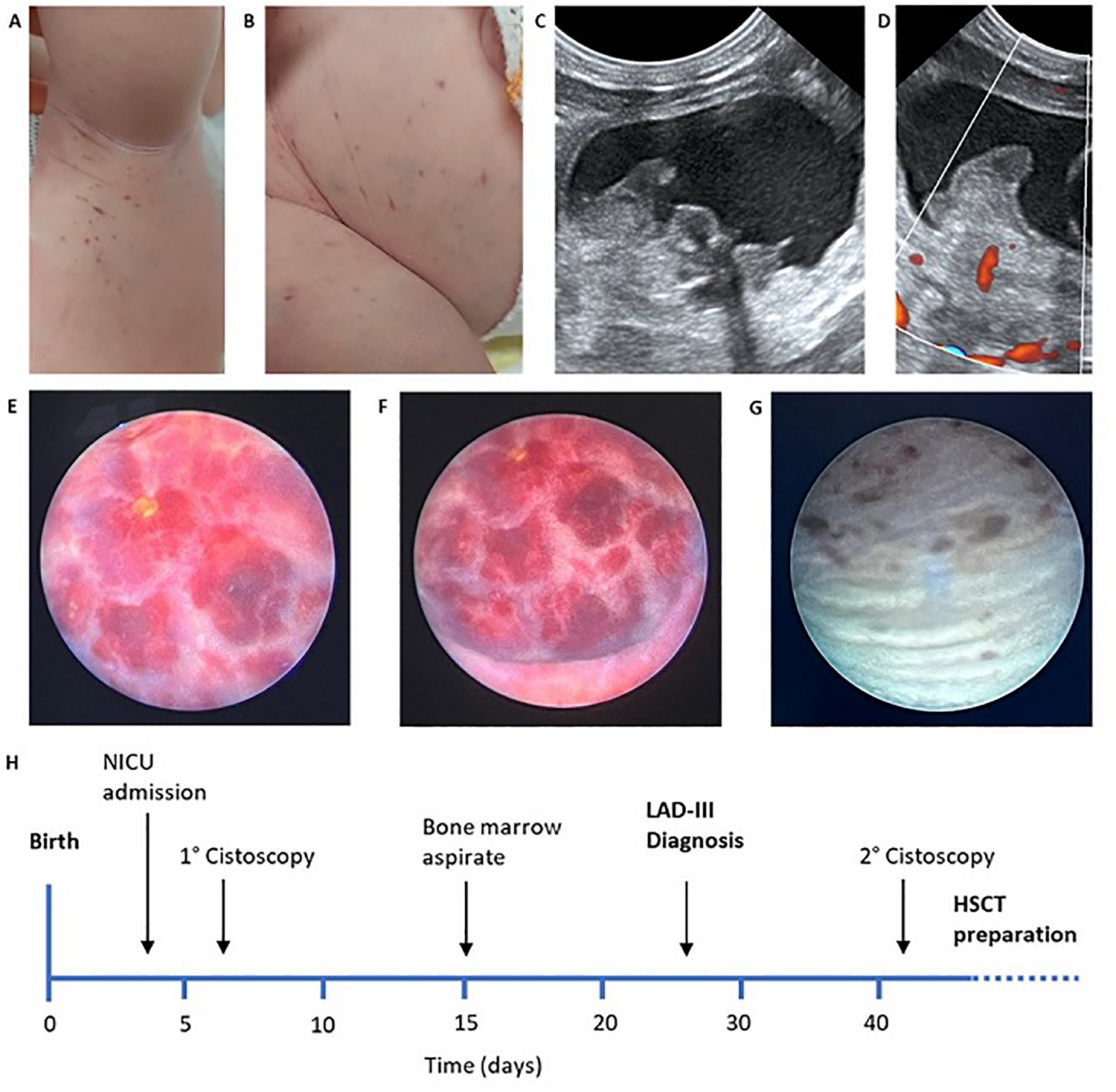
(A,B) Petechiae and ecchymoses over the trunk and limbs at admission; (C,D) early abdominal ultrasound showing vascularized endoluminal bladder wall mass; (E,F) early cystoscopy showing well demarcated hemangioma-like lesion of the bladder wall; (G) control cystoscopy after treatment showing almost complete resolution; (H) clinical history timeline.
